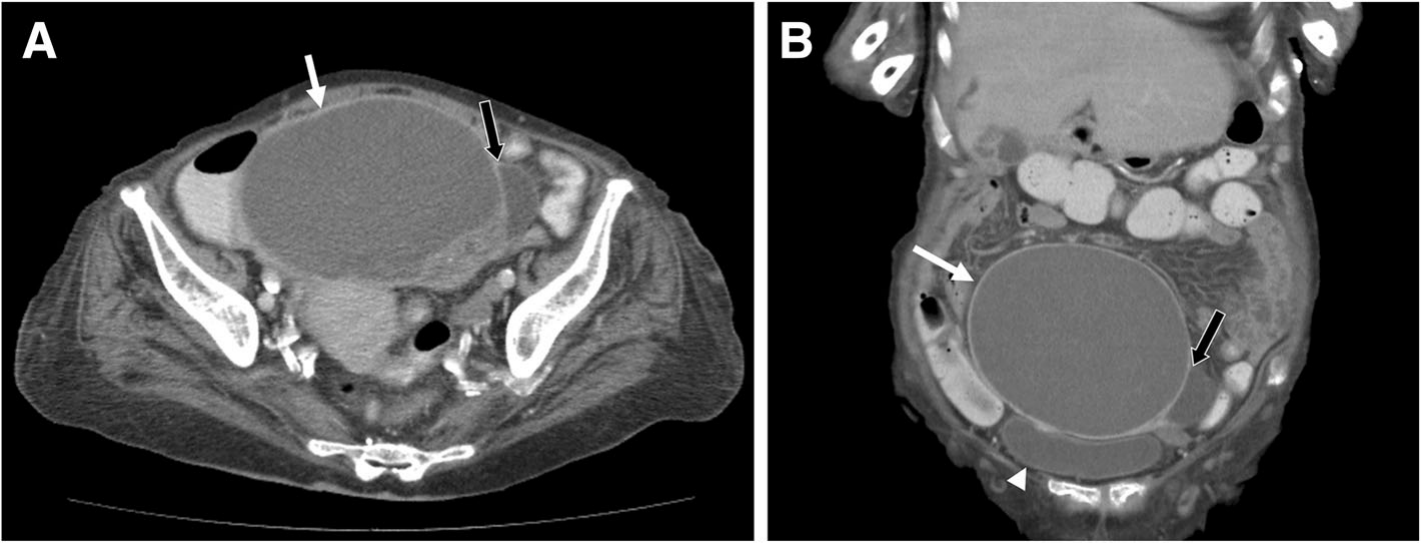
An abdominal computed tomography demonstrated the presence of a huge cystic mass 13.5 × 11.8 cm (white arrows) with internal septation (panel a, black arrows) and solid mural component. The urinary bladder (panel b, arrowhead) was compressed by it. No significant enlarged lymph nodes were found
